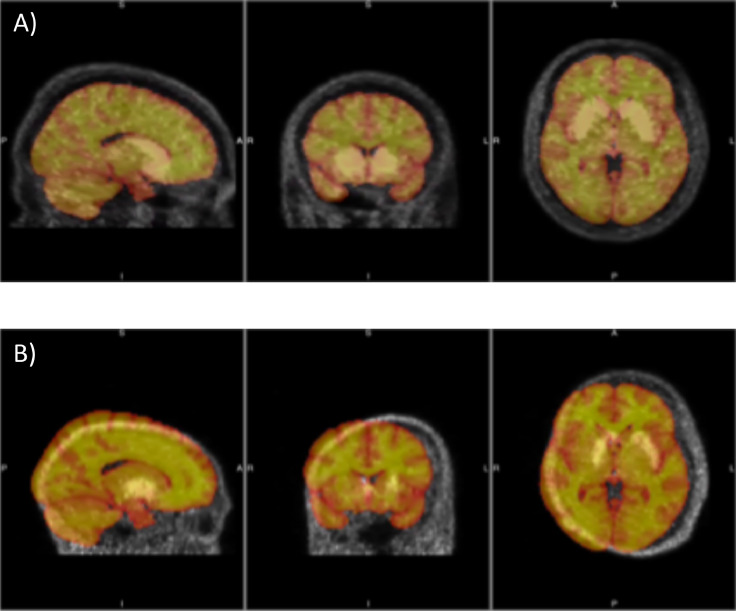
w Misalignment of FDOPA PET images to an MRI template. Example of aligned (a) and misaligned (b) [18F]-FDOPA PET images with an MRI template overlapped (orange). Sagittal (left), coronal (middle), axial al (right) views are shown.
